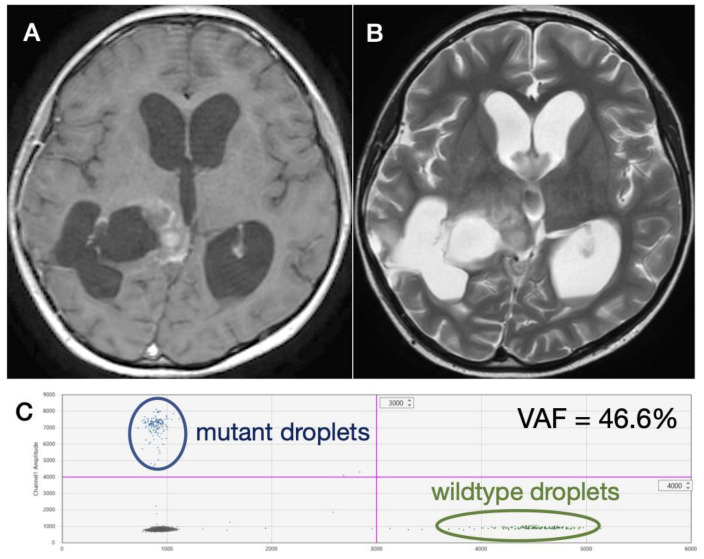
A recurrent DMG case in which *H3F3A* K27M was detected in CSF obtained during ventriculo-peritoneal shunting. MR images show (A) a heterogeneously enhancing residual lesion of the right thalamus and (B) enlarged ventricles. (C) Multiple *H3F3A* K27M-mutant droplets were detected by ddPCR analysis.
